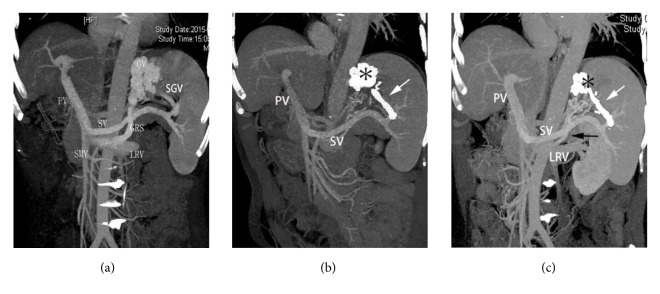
CT follow-up of the patient shown in Figure 2. (a) CT portal venography image obtained before percutaneous transhepatic antegrade variceal embolization showing large gastric varices with drainage by a large GRS. (b) CT portal venography image obtained 3 months after percutaneous transhepatic variceal embolization showing that the gastric varices (asterisk) and their feeding veins (white arrow) were filled with cyanoacrylate. (c) CT portal venography image obtained 1 year after percutaneous transhepatic variceal embolization showing that the cyanoacrylate in the submucosa varices had almost disappeared (asterisk), while the perifundus varices and the feeding veins (white arrow) were still filled with cyanoacrylate, similar to result observed before follow-up. Moreover, the gastrorenal shunt was reserved (black arrow). GRS, gastrorenal shunt; GV, gastric varices; SGV, short gastric vein; PV, portal vein; LRV, left renal vein; SMV, superior mesenteric vein; SV, splenic vein.
